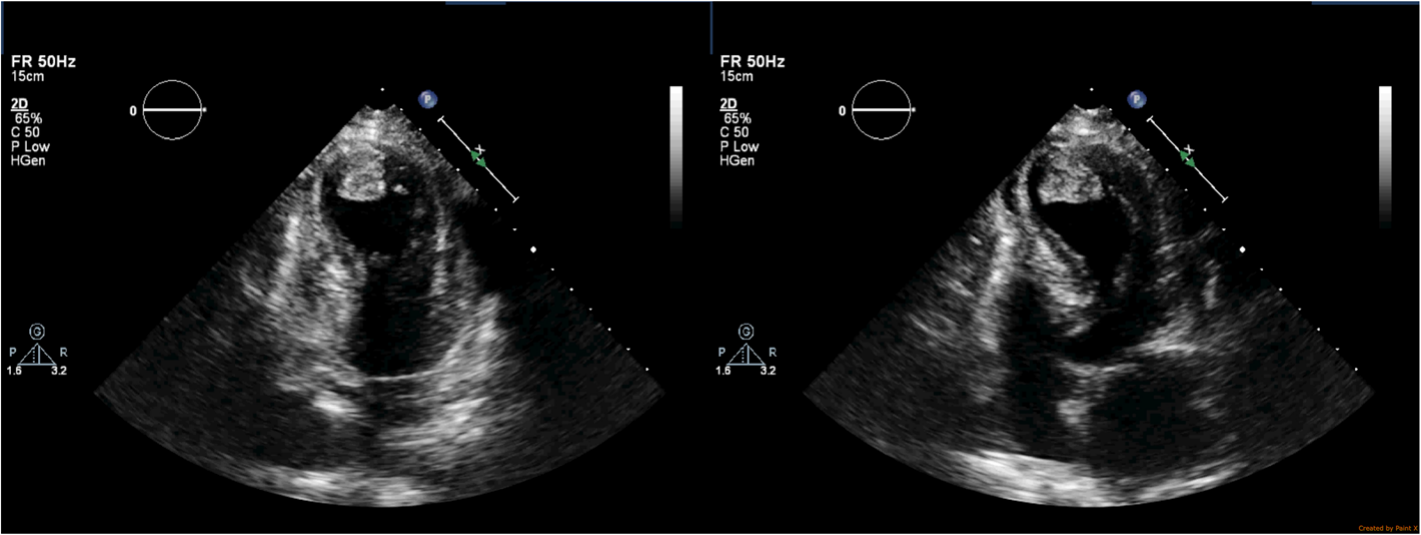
Transthoracic echocardiogram, performed 18 h after the onset of chest pain, showing hypokinesia of the *left* ventricular apex with apical ballooning. Ejection fraction was 40% and a 2.5 cm × 2 cm apical thrombus was detected
